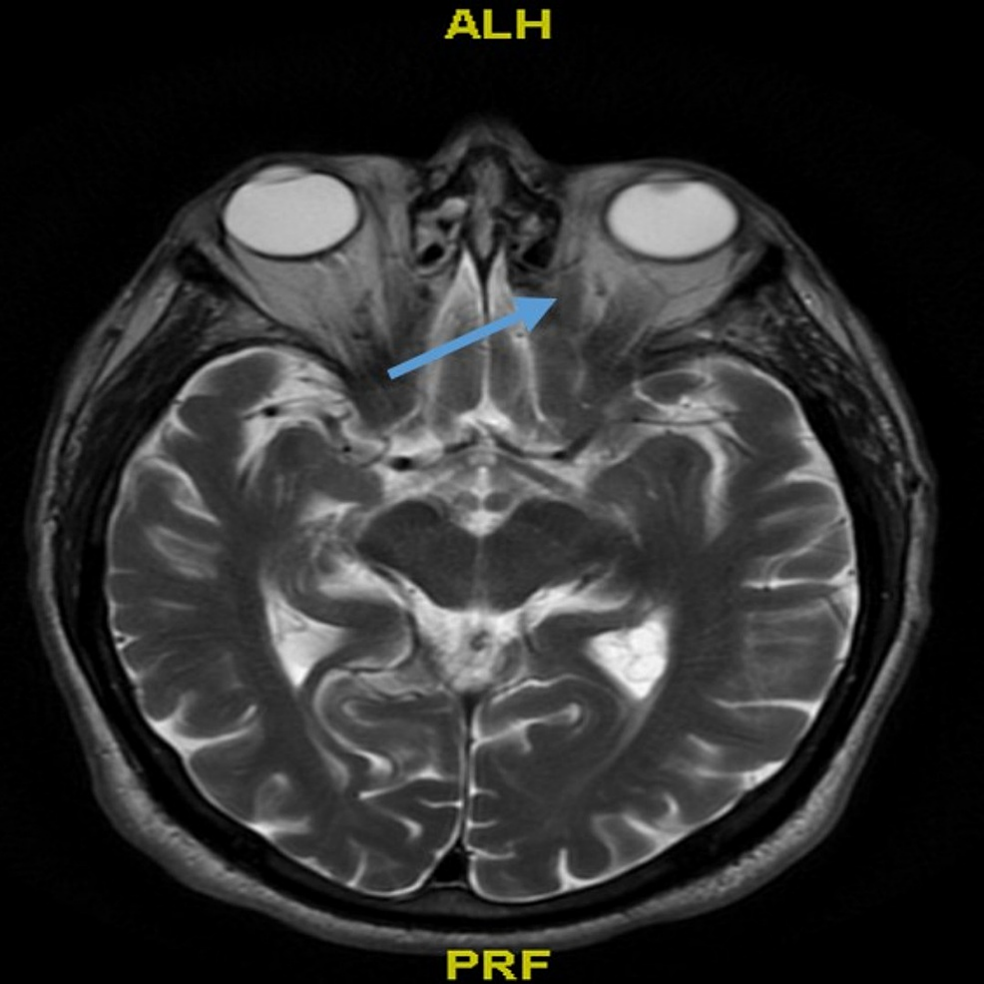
MRI brain with contrast The image shows extraocular myositis, which was more pronounced in the left medial rectus (blue arrow) MRI: magnetic resonance imaging
